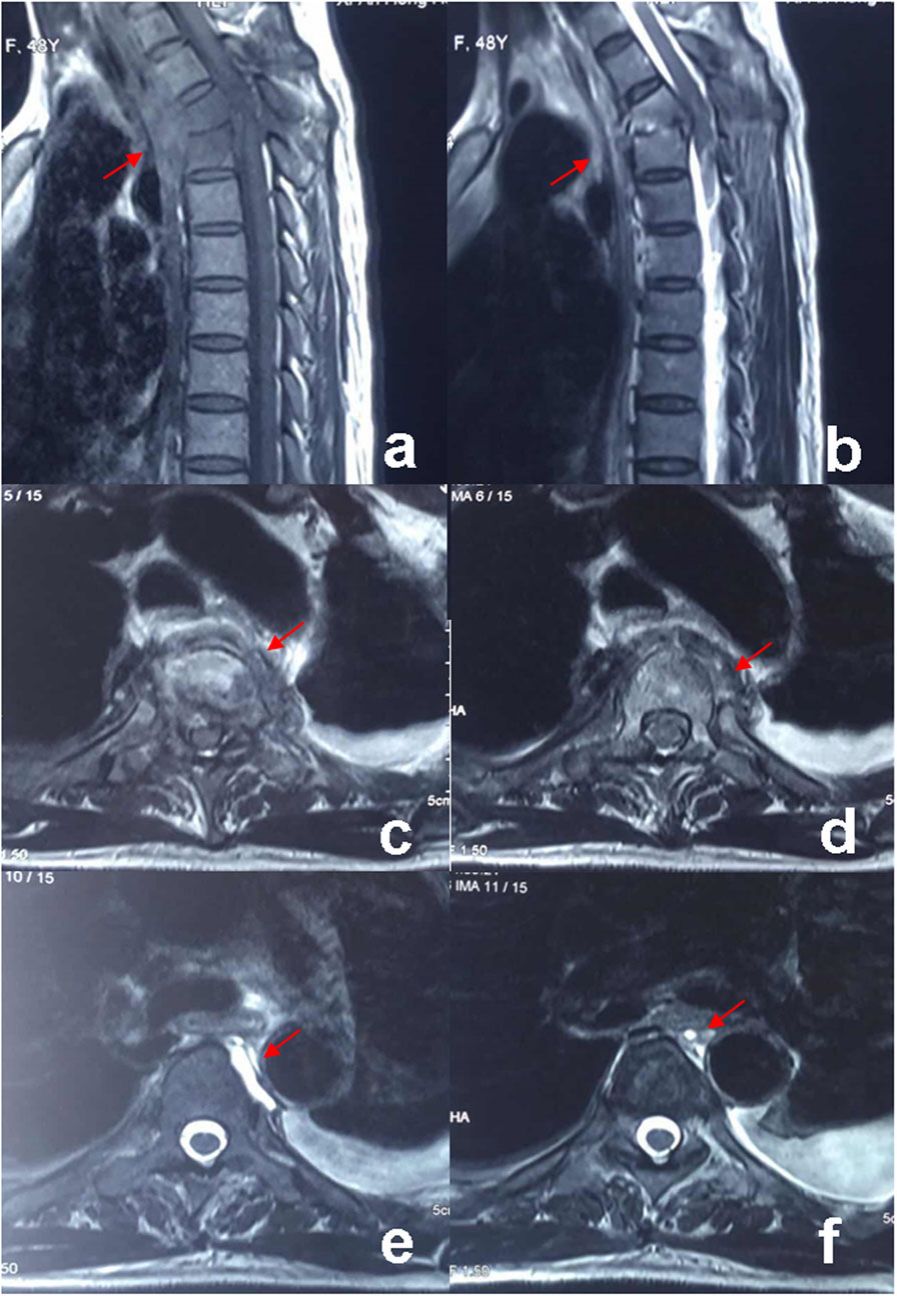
T1 (a) and T2 (b) sequences of MRI showed T3 / 4 vertebral infectious disease with spinal cord compression, and axial images (c-f) showed bone defects in the anterior part of T3 and T4 vertebrae, tuberculosis foci were located around the oesophagus and pushed the oesophagus to the right side (the red arrow indicates the location of the lesion)
